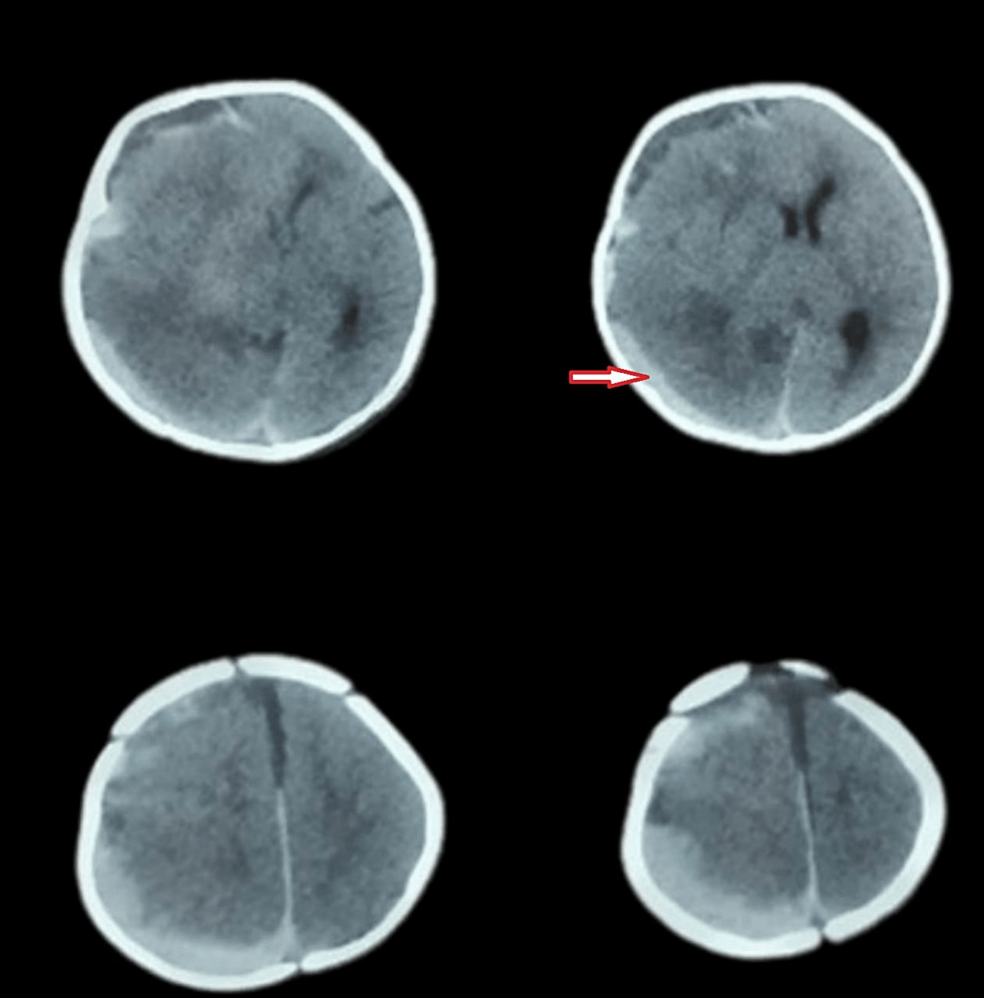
Radiological imaging on admission Orange arrow: CT brain showing subdural hematoma with mass effect CT: computed tomography
